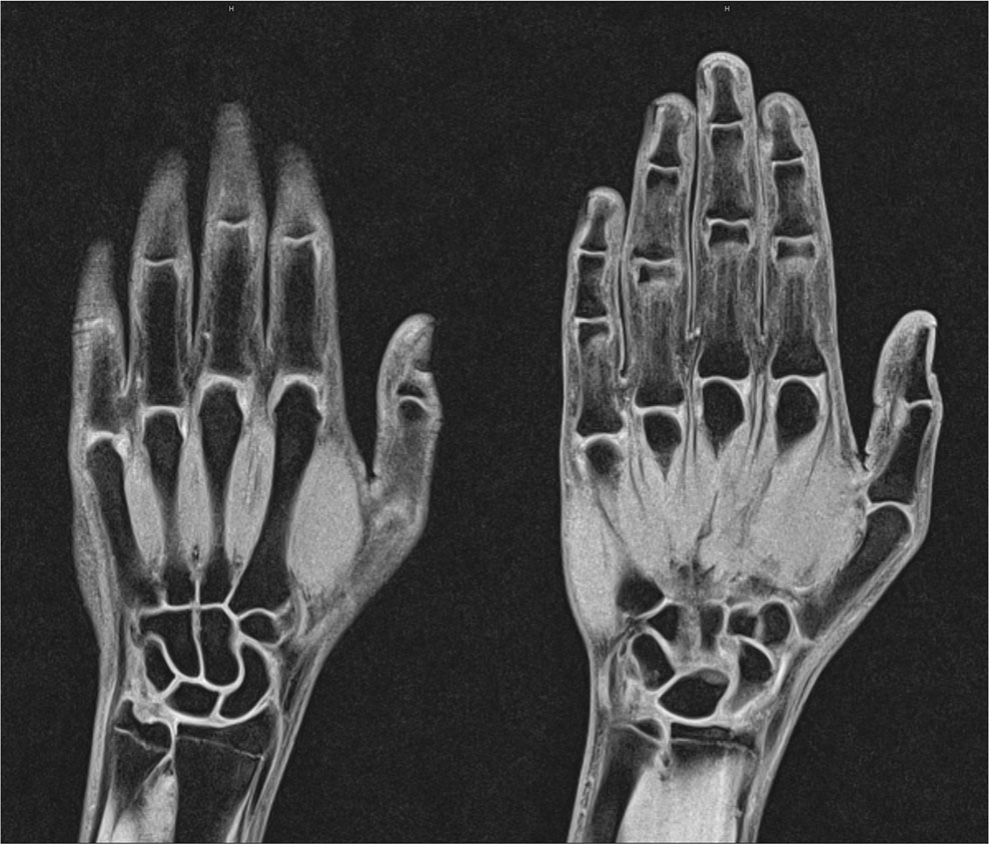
Two slices of a coronal T1 VIBE-3D-WE hand MRI of a 16-year-old healthy male. Estimated age was 17 years (reader 1) and 17 years (reader 2)
